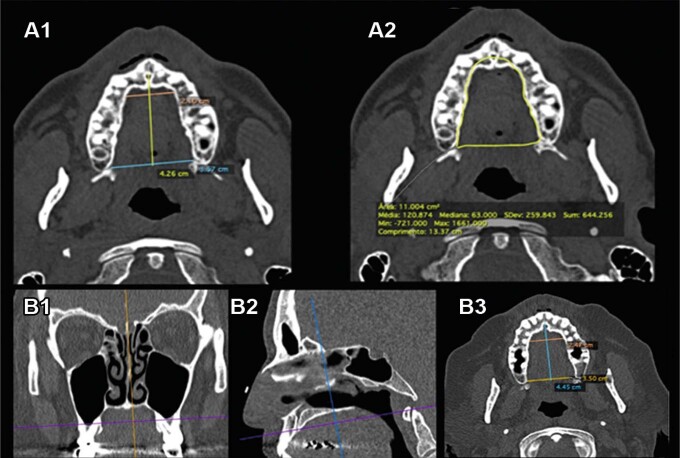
Demonstration of CT measurements of the palatal flap. A) Greatest anteroposterior distance, greatest transverse distance, area and perimeter; B) Alignment of cutting planes
